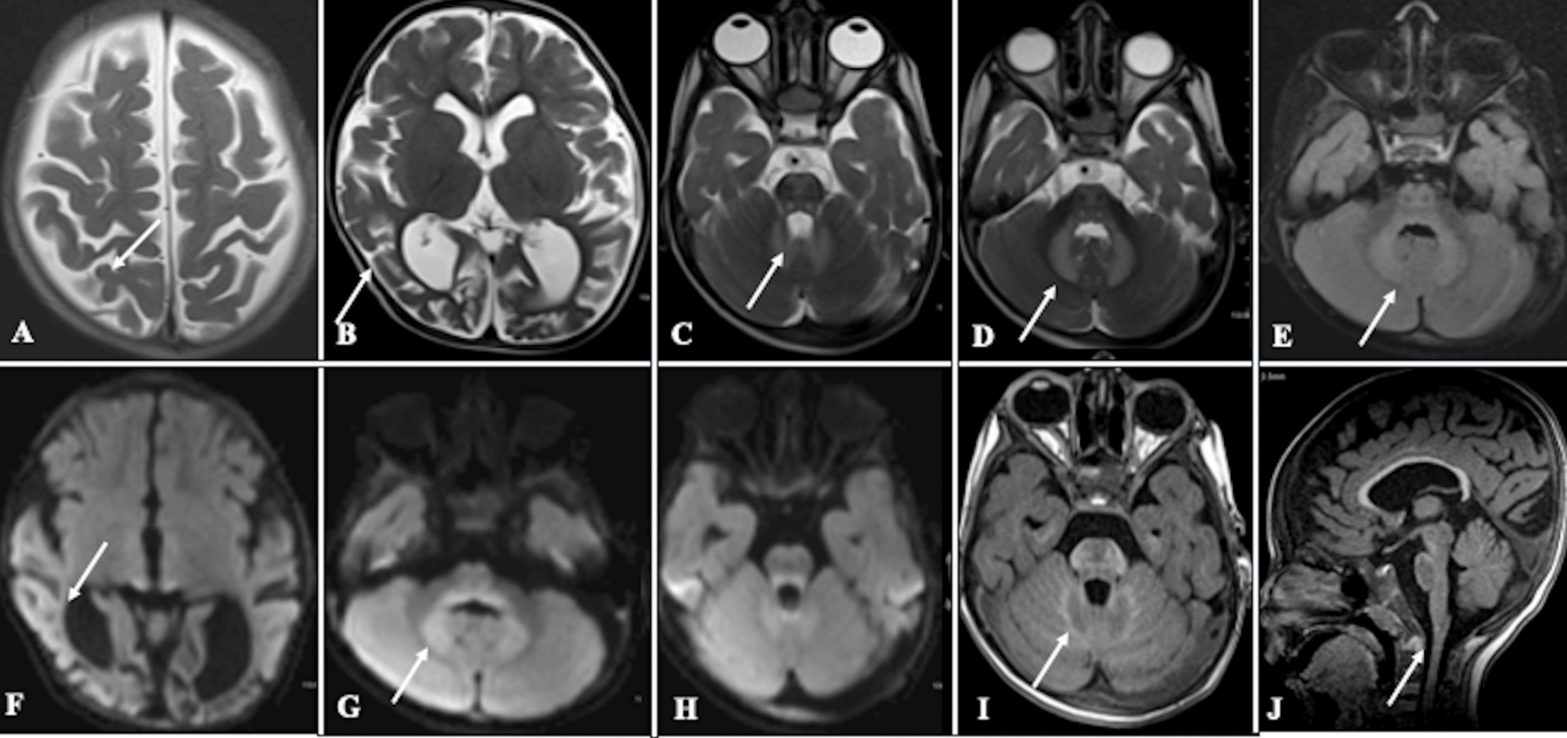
Magnetic resonance imaging (MRI) images of the brain showing the findings of EAST syndrome. Axial T2 (A-D) and Axial FLAIR (E) images showed cortical atrophy (white arrows in A, B) with hyperintense signal changes in cerebellar dentate nuclei, pons, and midbrain (white arrows in C-E) and restricted diffusion in the corresponding locations (white arrows in F-H). Axial T1 (I) showed the hypo intensity in the corresponding region (white arrow). Sagittal T1 (J) showed mild atrophy in the cervical spinal cord (white arrow). EAST: Epilepsy, Ataxia, Sensorineural Deafness, Tubulopathy Syndrome
